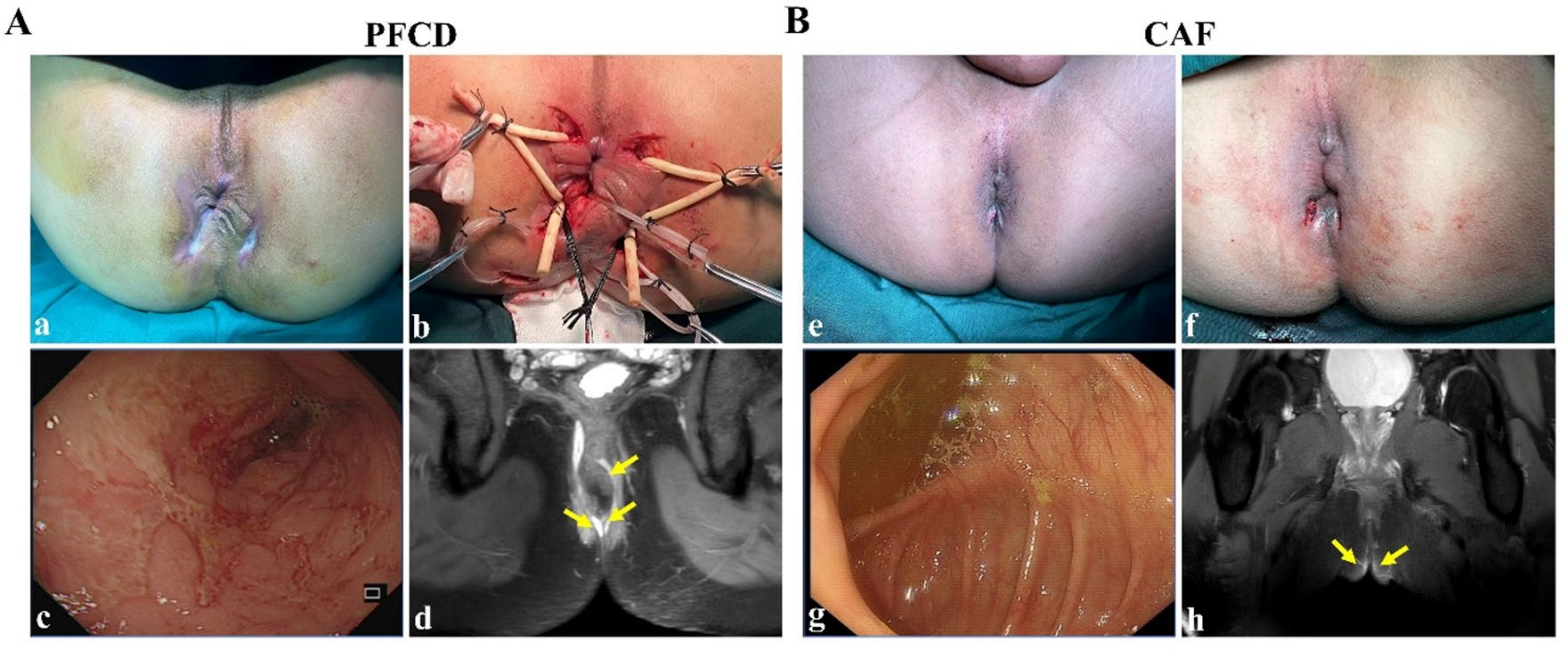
Clinical Characteristics of PFCD (A) and CAF (B). a, e: Pre-treatment of PFCD and CAF; b, f: Post-treatment of PFCD and CAF; c, g: Representative endoscopic picture captures of colon; d, h: perianal MRI (coronal T2WI-fat suppression), with fistula tracts (yellow arrow).
